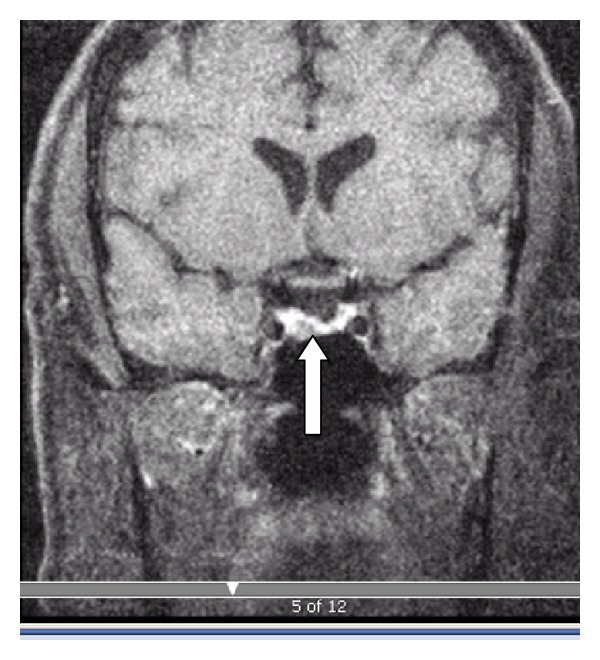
Pituitary MRI (post-contrast) showing microadenoma (thick arrow).
